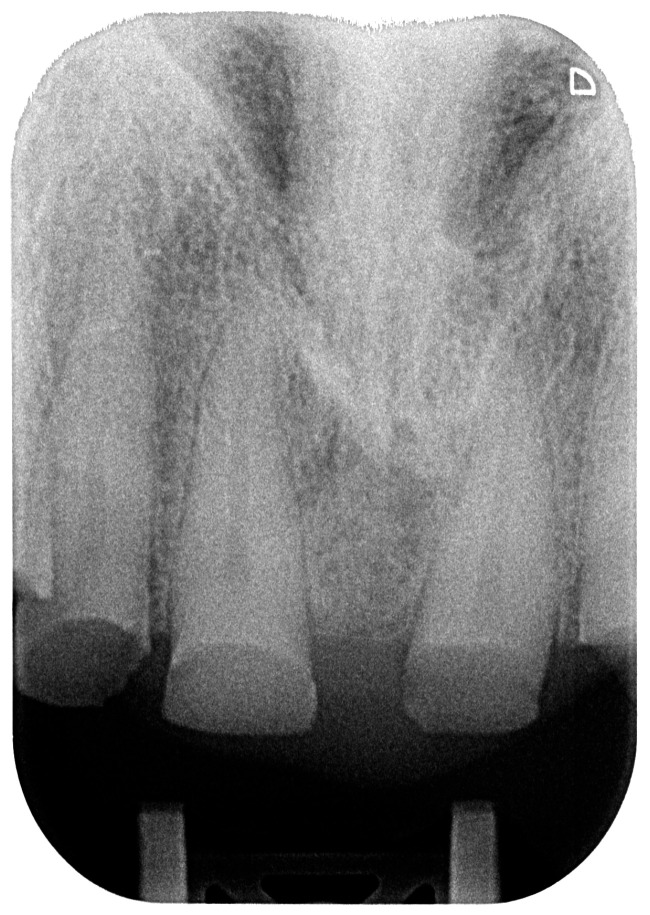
Initial radiographic status.
